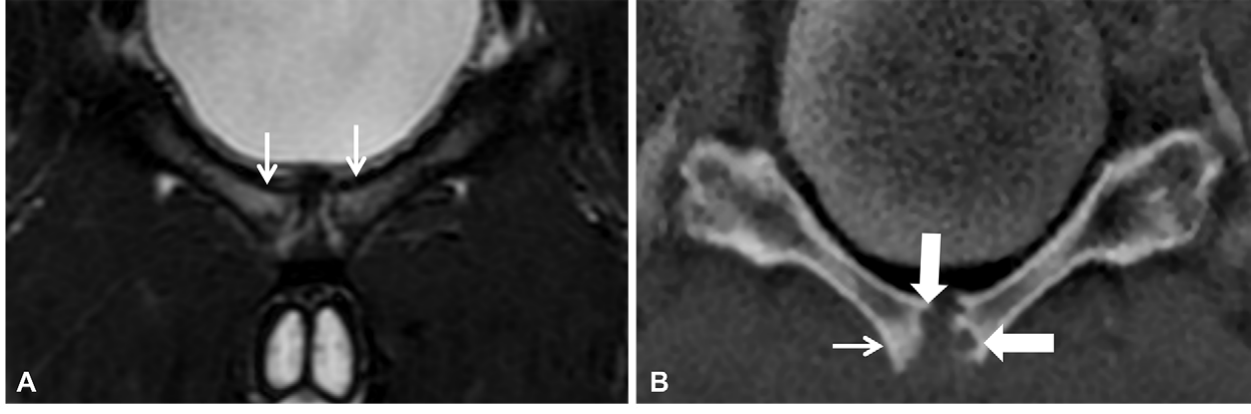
Coronal MRI of the pelvis in a 21-year-old male sub-elite football player demonstrating pubic apophysitis. (A) The coronal T2 fat-suppressed image demonstrates bilateral bone marrow edema at the pubic symphysis, more pronounced on the right (arrows). (B) The coronal ZTE image demonstrates widening of the pubic symphysis with adjacent sclerosis (arrow) and focal irregular delineation of the articular margins (bold arrow).
